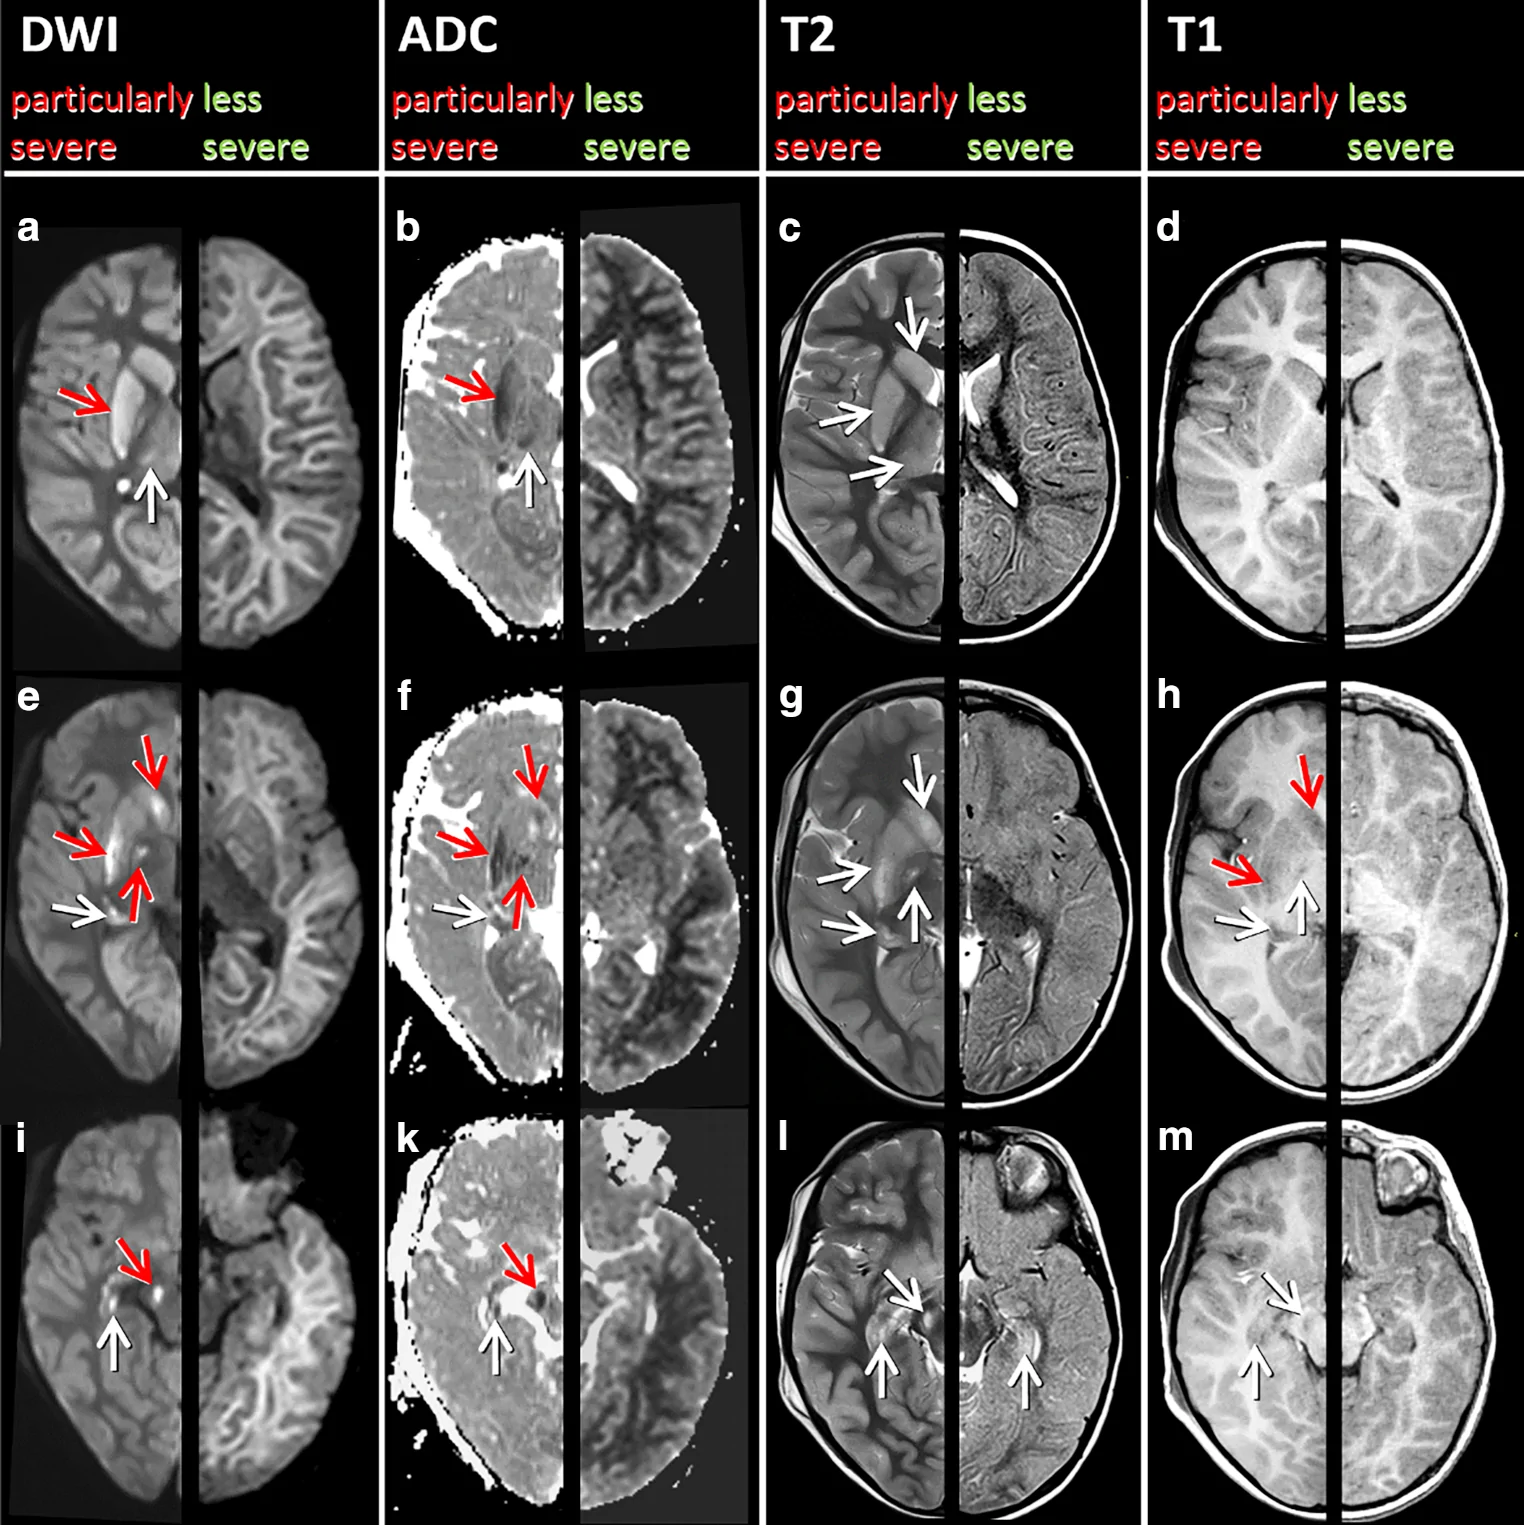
MRI obtained 4–5 days after the anoxic event. Structures with abnormal signal intensities are marked with arrows (red/dark gray = predicting particularly severe outcome). Particularly severe outcomes could be predicted by diffusion abnormalities in the globus pallidus (red/dark gray arrows in e,f), in the substantia nigra (red/dark gray arrows in i,k) and in the putamen and the caudate nucleus (red/dark gray arrows in a,b,e,f). Particularly severe outcomes could furthermore be predicted by T1-hypointensities in the putamen and the caudate nucleus (red/dark gray arrows in h). Note that diffusion abnormalities began to occur now also in the cerebral white matter in some patients (a,b,e,f,i,k—right sides), however this was not yet predictive. Note the—also non-predictive—characteristic appearance of cortical laminar necrosis [34] in c,g,l—right sides
